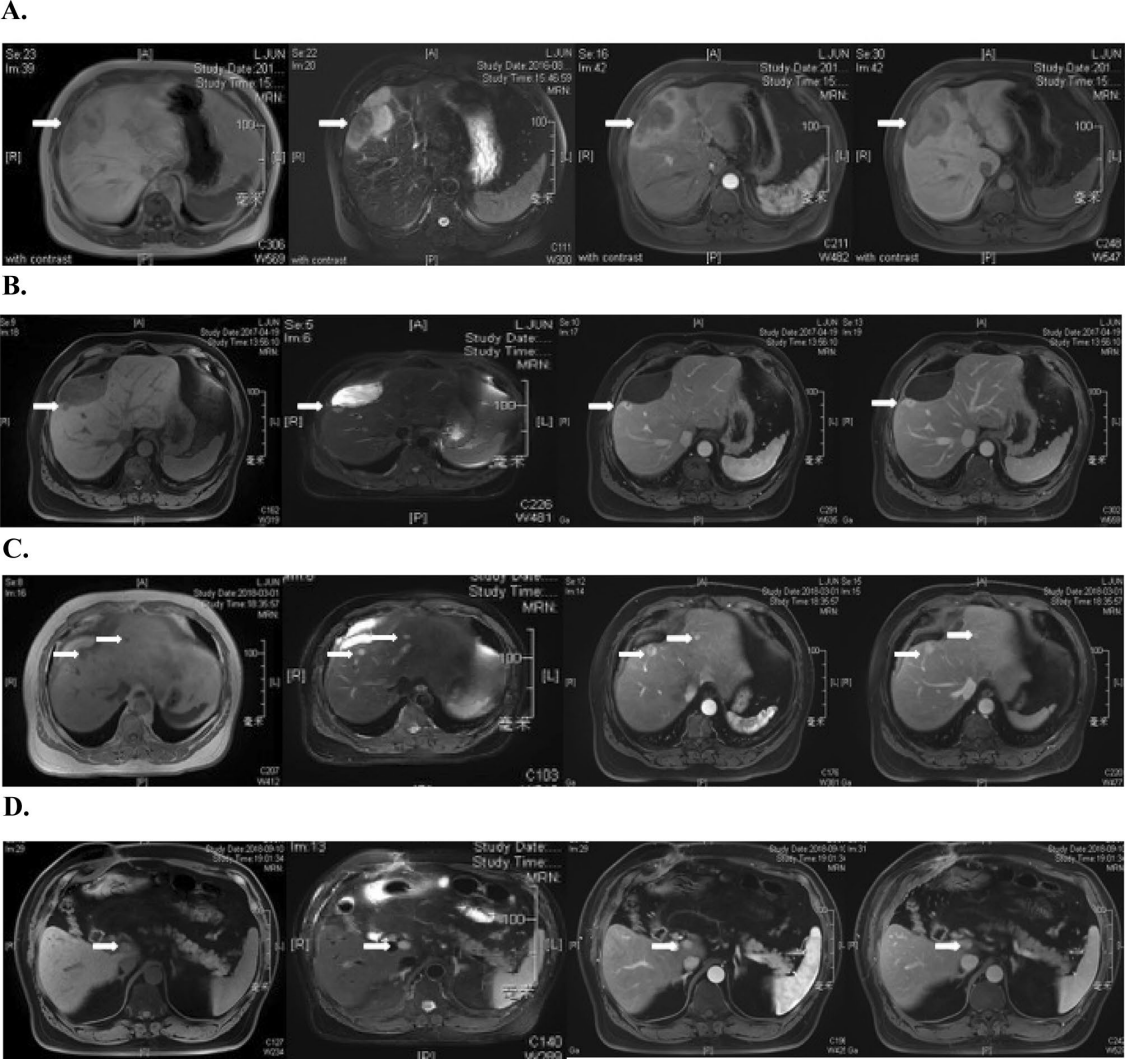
Enhanced abdominal magnetic renounce imaging (MRI) of the reported case. a The white arrow heads a hypovascular liver lesion (9.5 cm). b The white arrow directs to the recurrent lesion (about 1 cm) adjacent to the first operation zone (1st recurrence). c The white arrow shows the recurrent tumors in liver after re-resection (2nd recurrence). d The white arrow pointed to the liver lesion after open-surgical microwave ablation (3rd recurrence)
